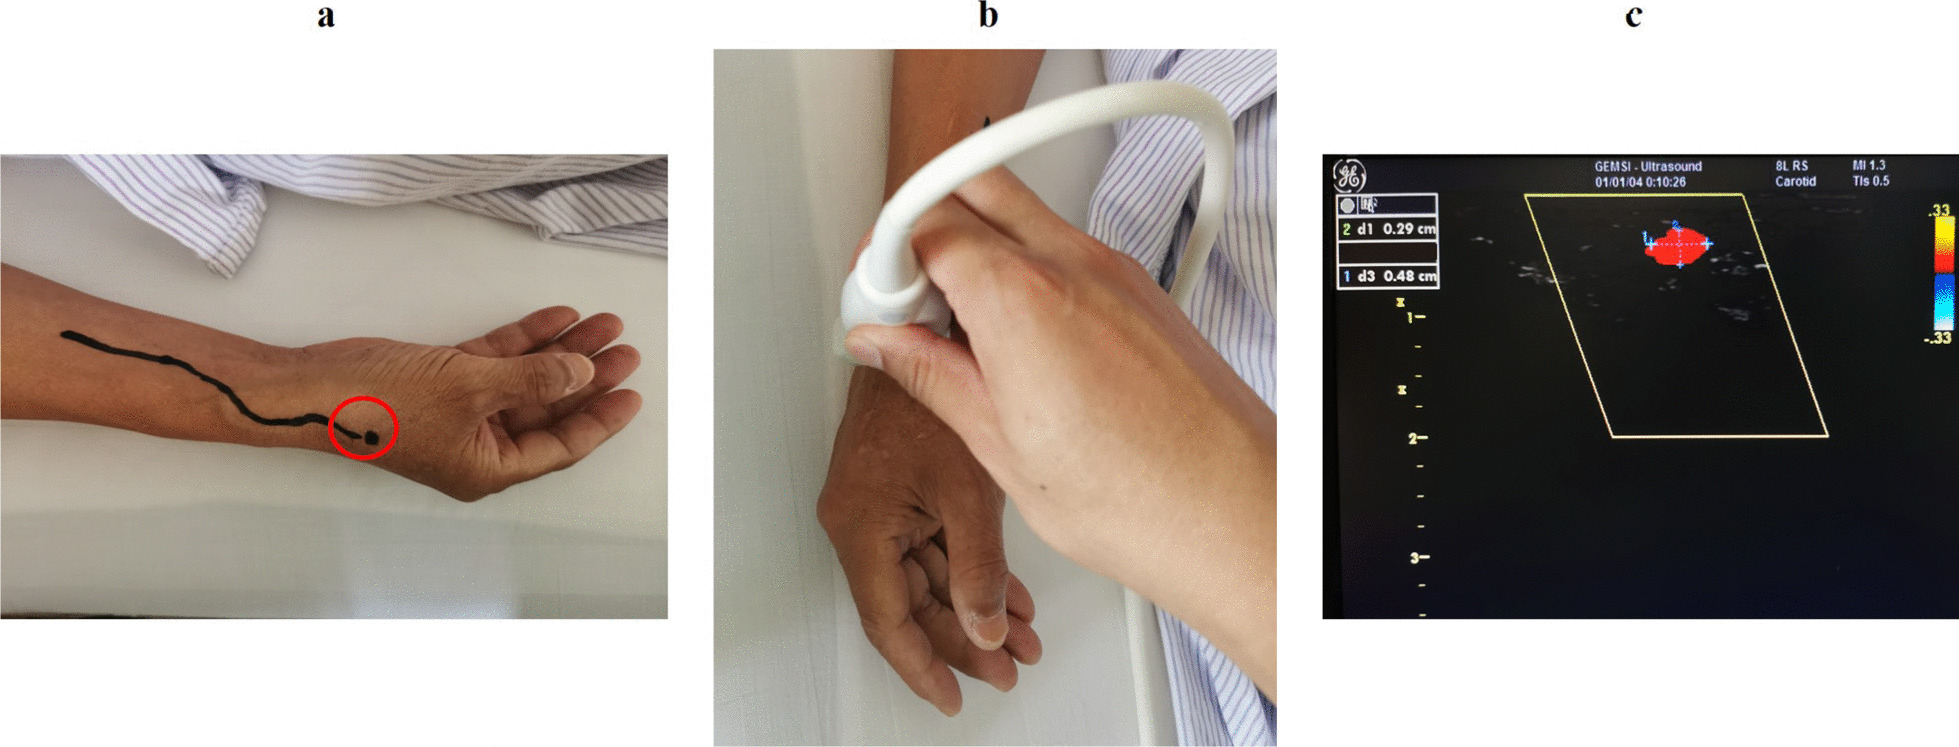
Identification of the superficial radial artery under the guidance of ultrasonography. a The course of the superficial radial artery (black line) and the puncture site of the distal radial artery (black dot) in the anatomic snuffbox (red circle) were marked. b The assessment of the distal radial artery condition. c The diameter of the distal radial artery lumen was 0.29 × 0.48 cm
